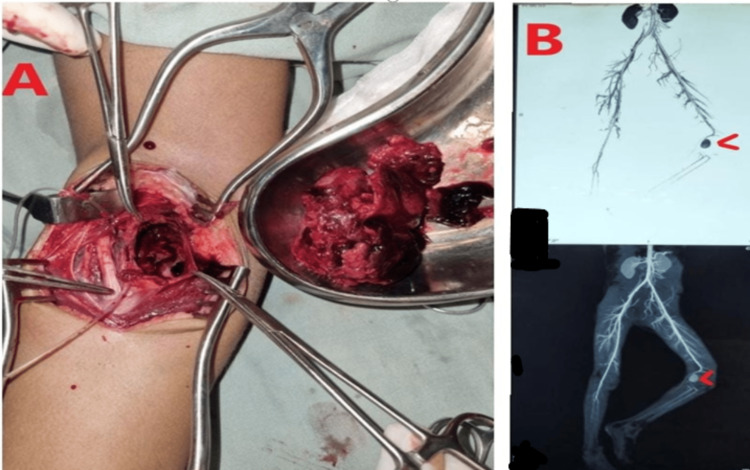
A: Aneurysm of the popliteal artery in situ. B: Isolated aneurysm on CT scan with and without contrast. CT: computed tomography
